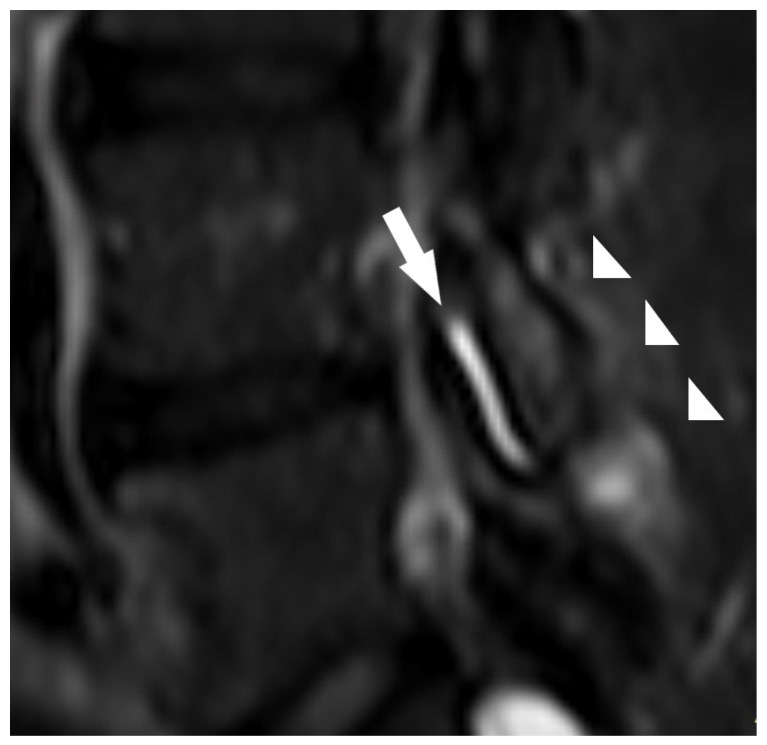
Magnetic resonance image showing the LBLP case with facet effusion (arrow), bone marrow, and surrounding soft-tissue edema (arrowheads).
